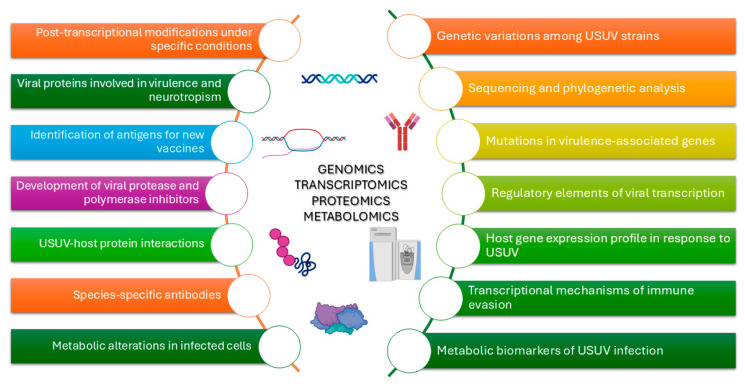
Application of genomics, transcriptomics, proteomics, and metabolomics in USUV research. This image highlights various aspects of analysis, including gene expression, sequence variations, pathogenic mechanisms, immune response, and viral strain typing, emphasising the importance of a multidisciplinary approach to understanding USUV transmission, pathogenesis, and potential control strategies.
